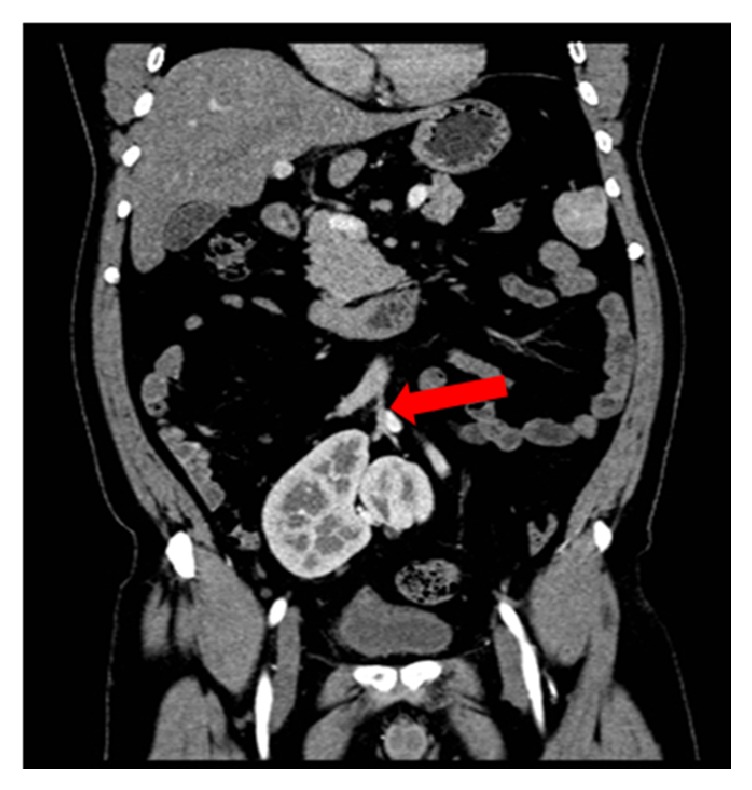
Coronal CT scan with IV contrast showing the median sacral artery (red arrow) giving right and left renal arteries.
